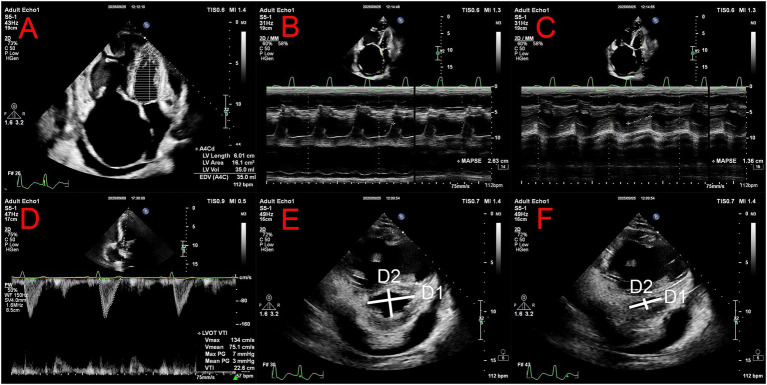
Assessment of left ventricular in pulmonary hypertension. (A) Left ventricular end-diastolic volume measurement in the apical four chamber view. (B) Septal MAPSE measurement in the apical four chamber view. (C) Lateral MAPSE measurement in the apical four chamber view. (D) The velocity time integral of the left ventricular outflow tract measured by pulsed-wave Doppler. Eccentricity index, the ratio of the diameters (from compact myocardium) parallel (D1) and perpendicular (D2) to the ventricular septum, as measured at end diastole (E) and end systole (F).
